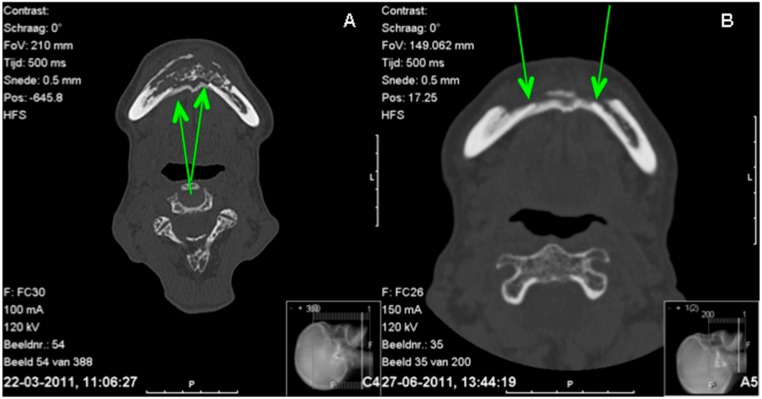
Comparison CT scans before (A) and three months after surgery (B). A = lingual subperiosteal bone can be seen and seems to connect both parts of the mandible; B = the difference between the cortex of the mandible and the subperiosteal bone is decreasing.
